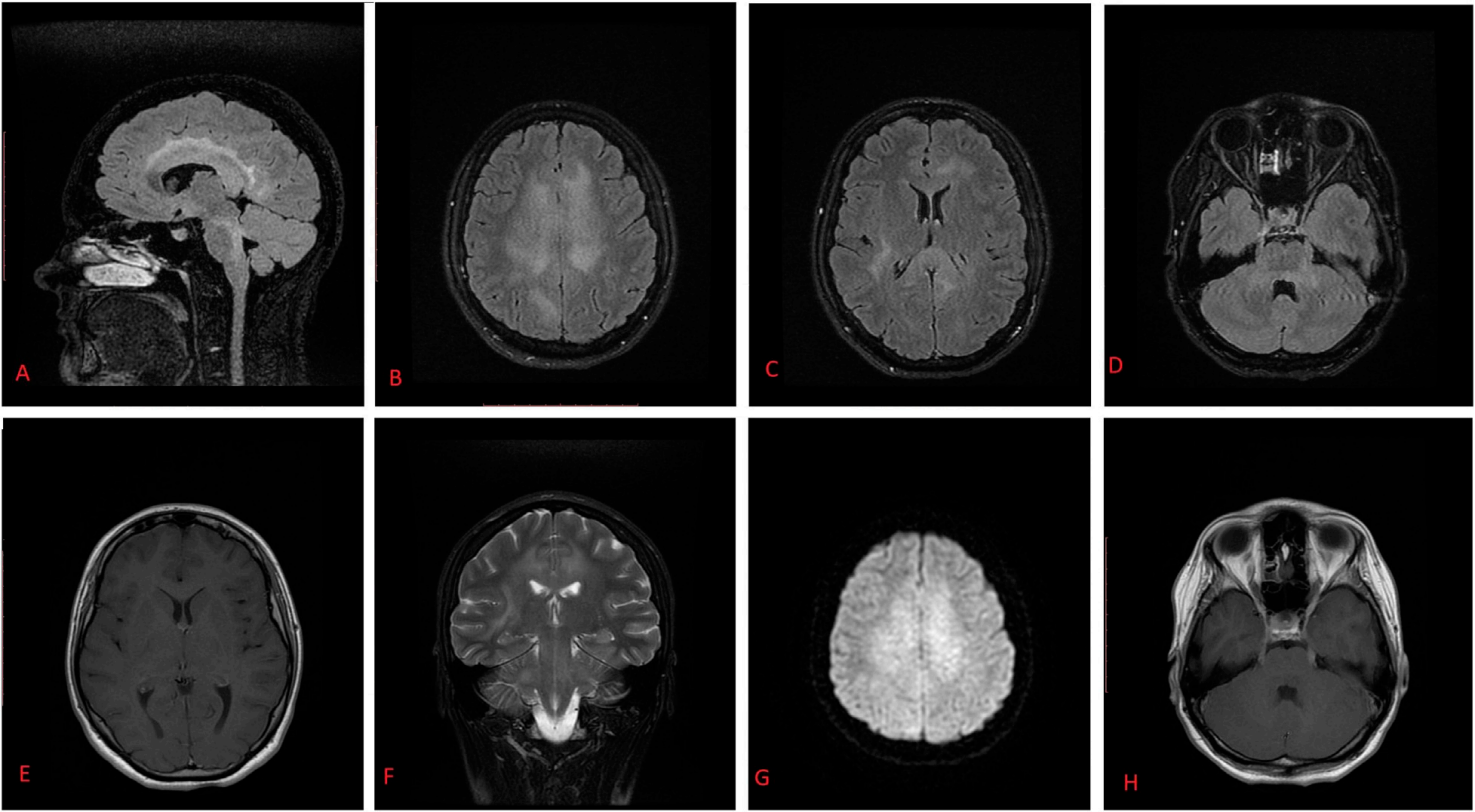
T2 FLAIR MR images show hyperintense right corticospinal tract (A), corona radiata, frontal and parietal lobes signal (B), corpus callosum dorsal parts (C), cerebral peduncular, and hemisphere white matter (D,F). Contrast-enhanced T1-weighted axial MR image shows no visible contrast enhancement or intraaxial changes (E). Diffusion-weighted axial MR images show slightly restricted diffusion (G). Contrast-enhanced T1-weighted axial MR images show enlargement of cranial nerves bilaterally (n.oculomotorius, and n. trigeminus) (H). The differential of these MR findings included viral encephalitis, demyelinating disease, lymphoma, and leptomeningeal metastases.
